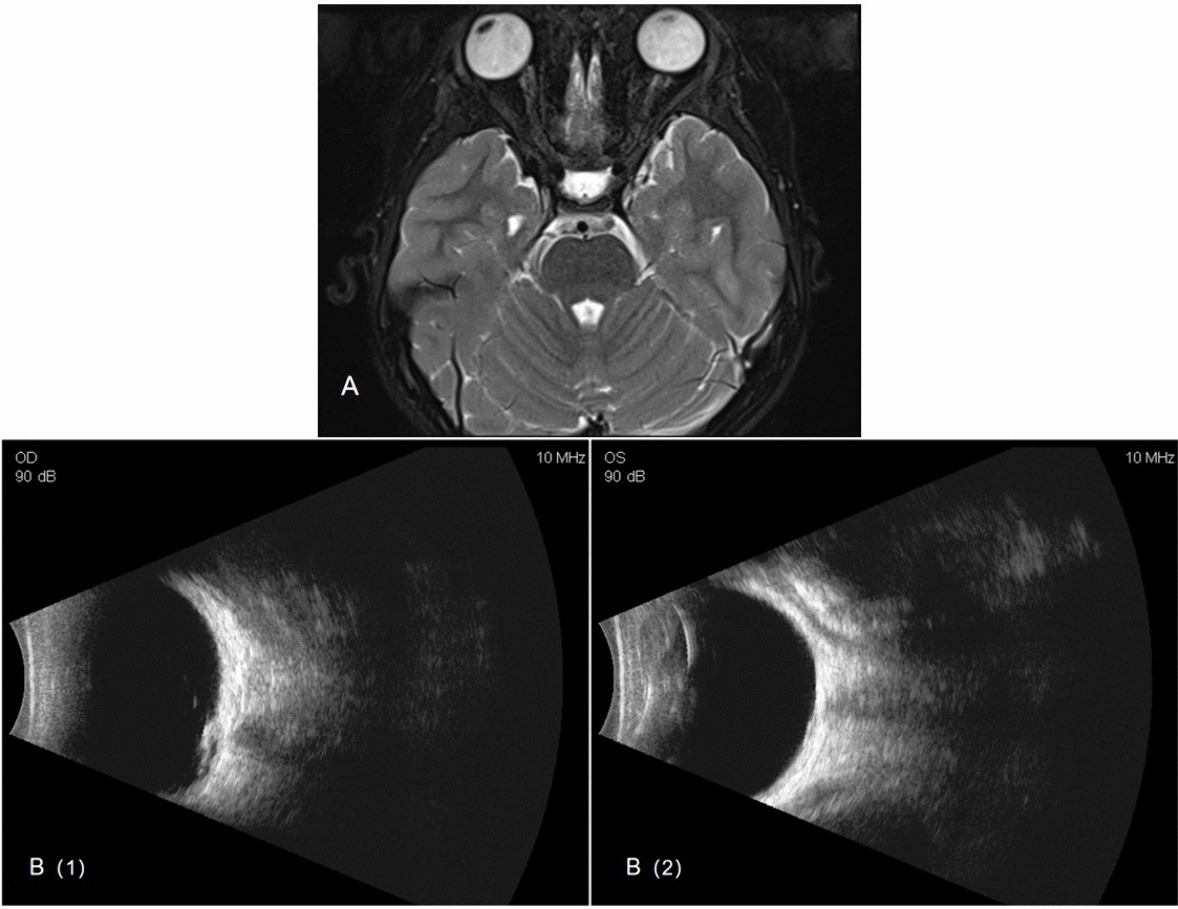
MRI (A) and B-ultrasound (B) of the eye showing a single optic nerve connected to both eyes
